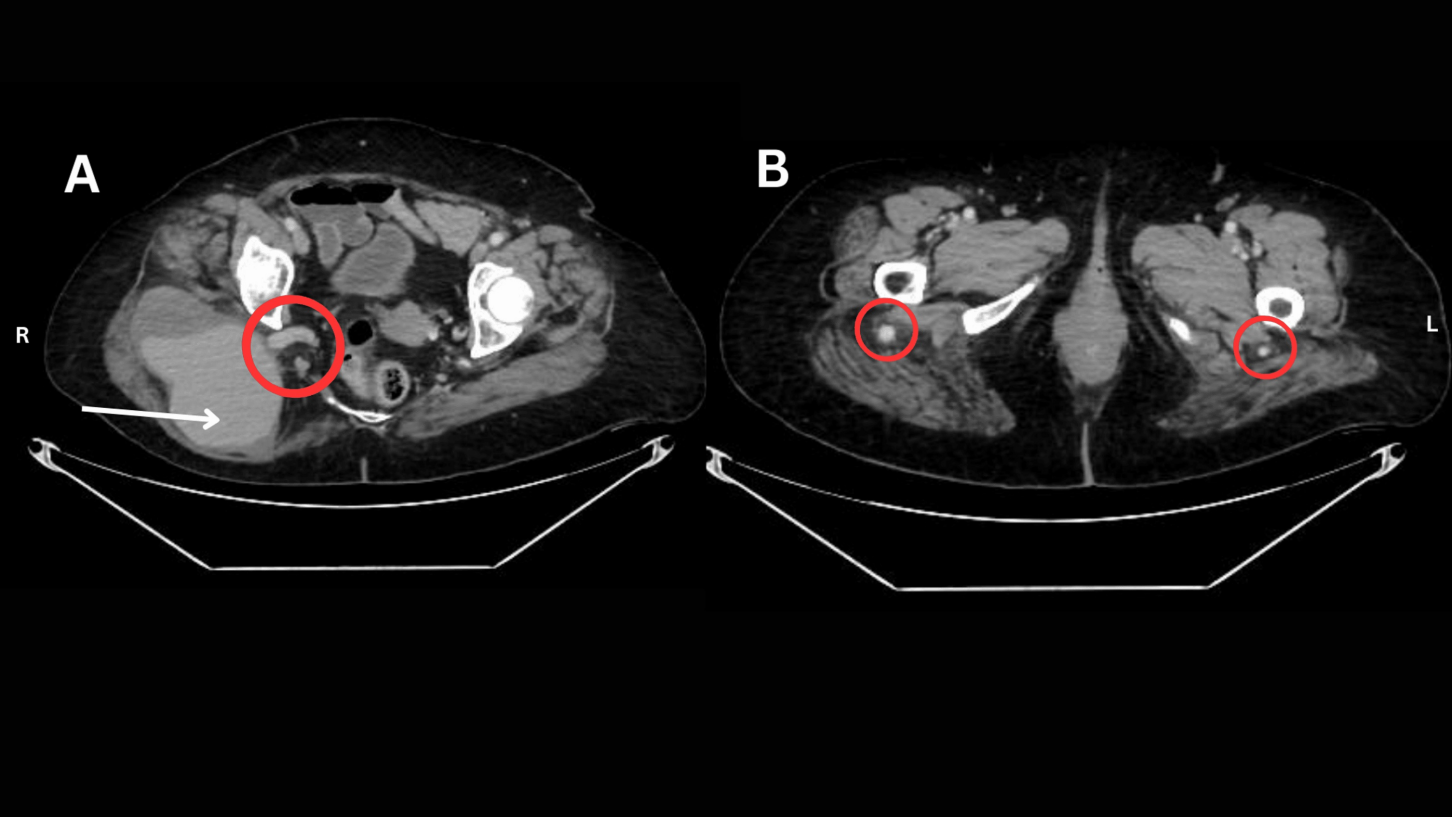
A) Axial CT angiogram at the upper gluteal region image: red circled region depicts the origin of the right PSA from the right internal iliac artery. The white arrow indicates a large PSA aneurysm measuring 11cm X 7cm with partial thrombosis. B) Axial CT angiogram at the lower gluteal region showing bilateral PSAs, with the left PSA displaying a relatively reduced luminal diameter compared with the right.
